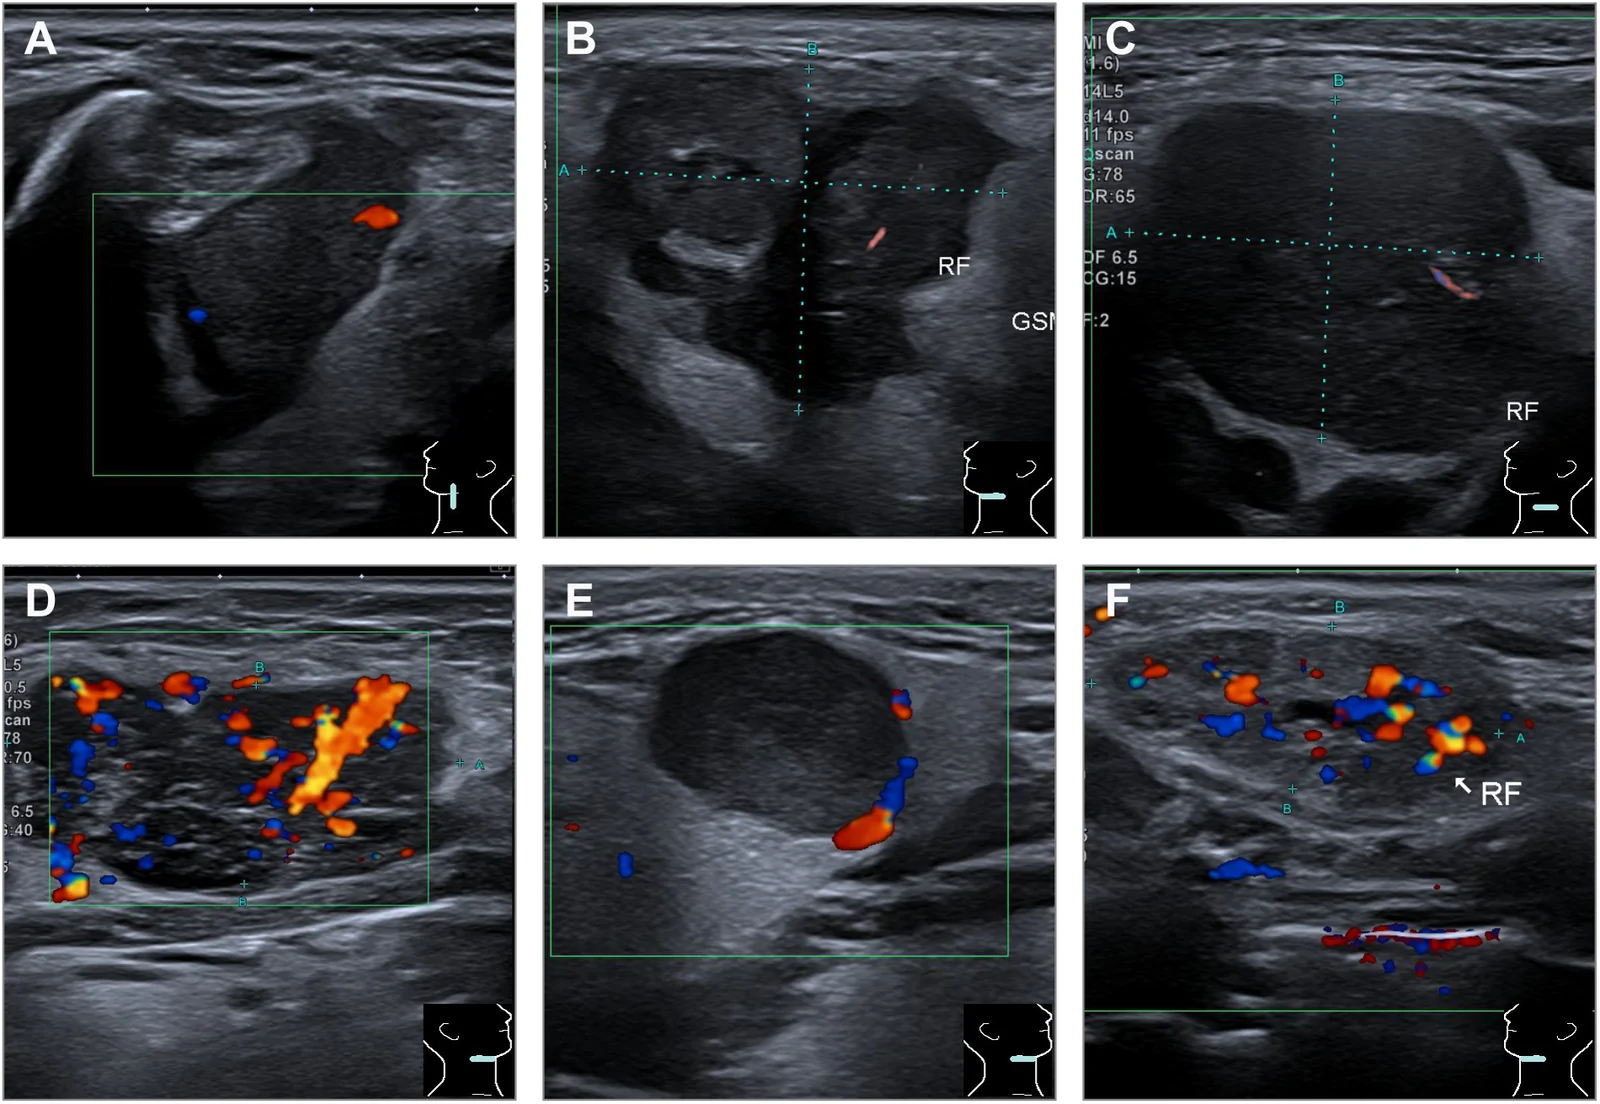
Color Doppler sonographic images (PLT-1005BT, 10 MHz, Aplio i800 and Aplio a, canon medical systems); added pictogram in the bottom right indicating scan plane and side. (A) Adenoid cystic carcinoma, (B) salivary duct carcinoma, (C) a highly suspicious cervical lymph node in level II of salivary duct carcinoma, (D) follicular intraglandular lymphoma (B-cell non-Hodgkin lymphoma), (E) pleomorphic adenoma, and (F) Küttner tumor (chronic IgG4-positive sclerosing sialadenitis).
